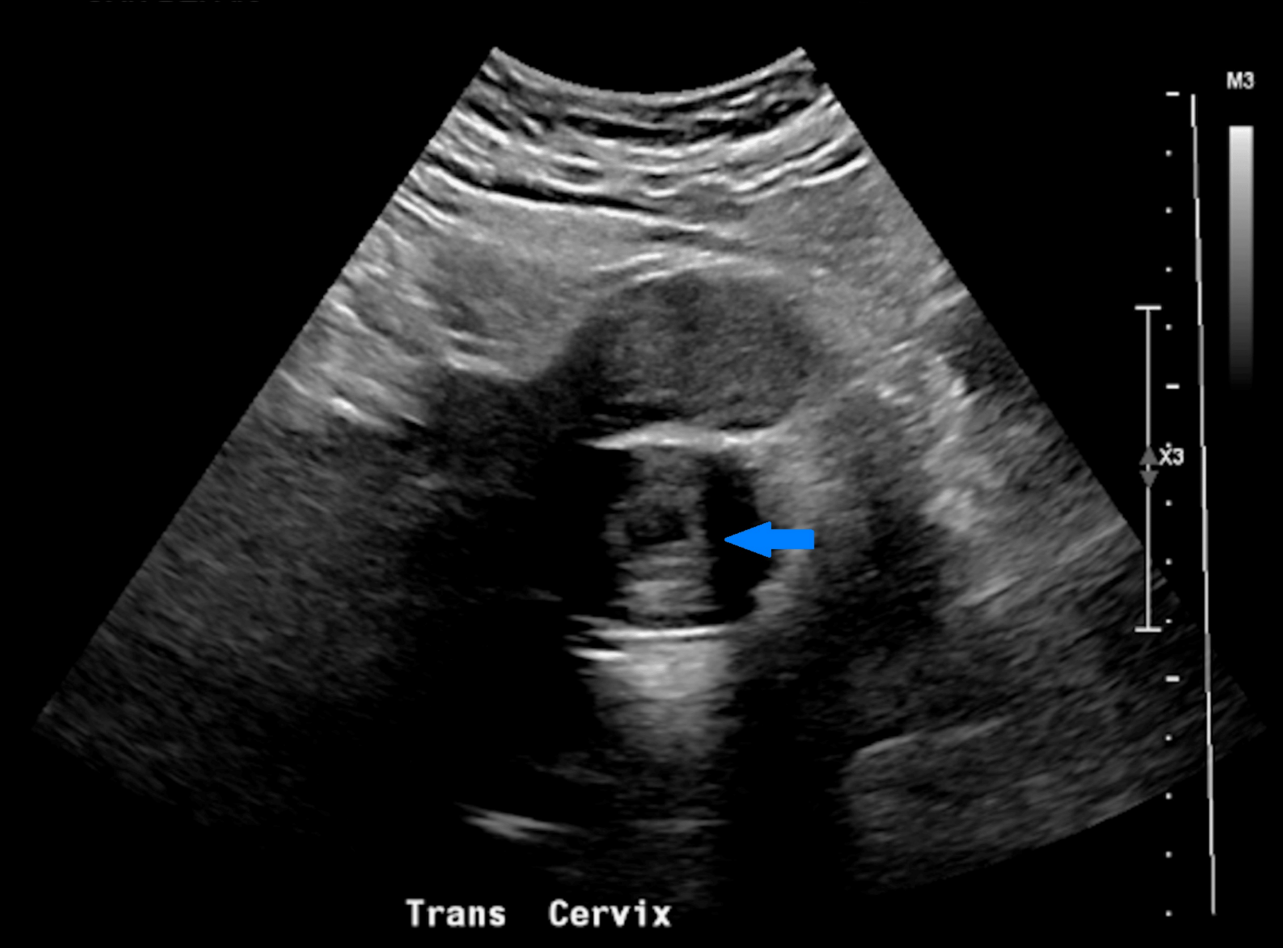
Transabdominal ultrasound on Day 0 demonstrating cervical ectopic pregnancy. Transabdominal ultrasound performed on Day 0 demonstrates a gestational sac implanted within the cervical canal (blue arrow), below the level of the internal os, consistent with a cervical ectopic pregnancy.
